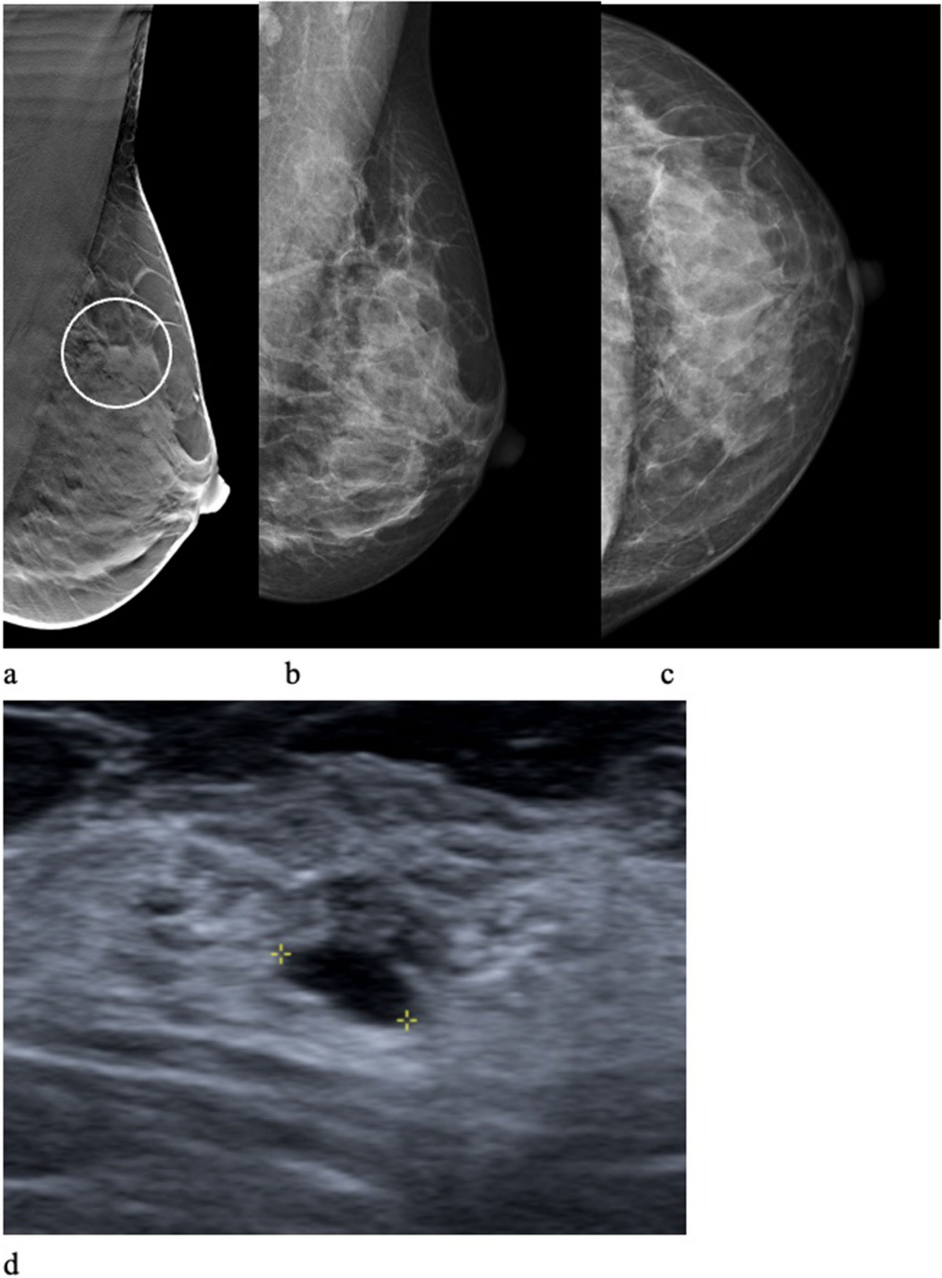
A 49-year-old woman was recalled in the DBT group due to a circumscribed mass (circle). One-view digital breast tomosynthesis (a) and mediolateral oblique (b) and craniocaudal (c) views at digital mammography at screening. Ultrasound (d) showed a benign cyst confirmed by fine needle aspiration
